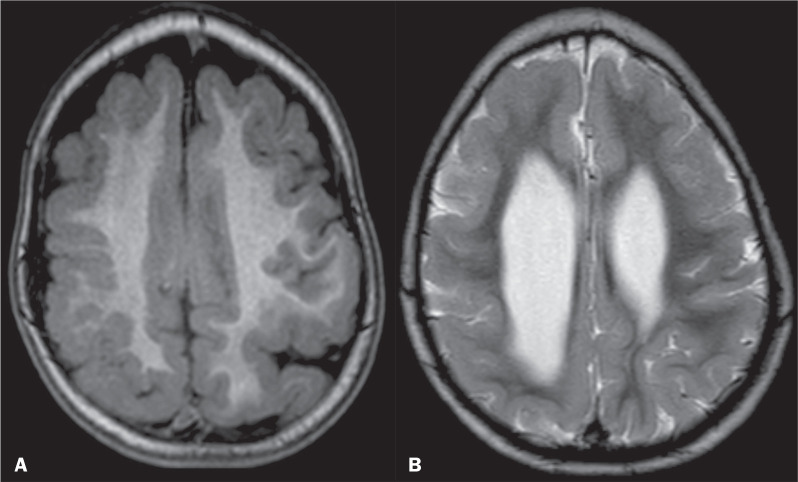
Malformations of cortical development on MRI in a 19-monthold
